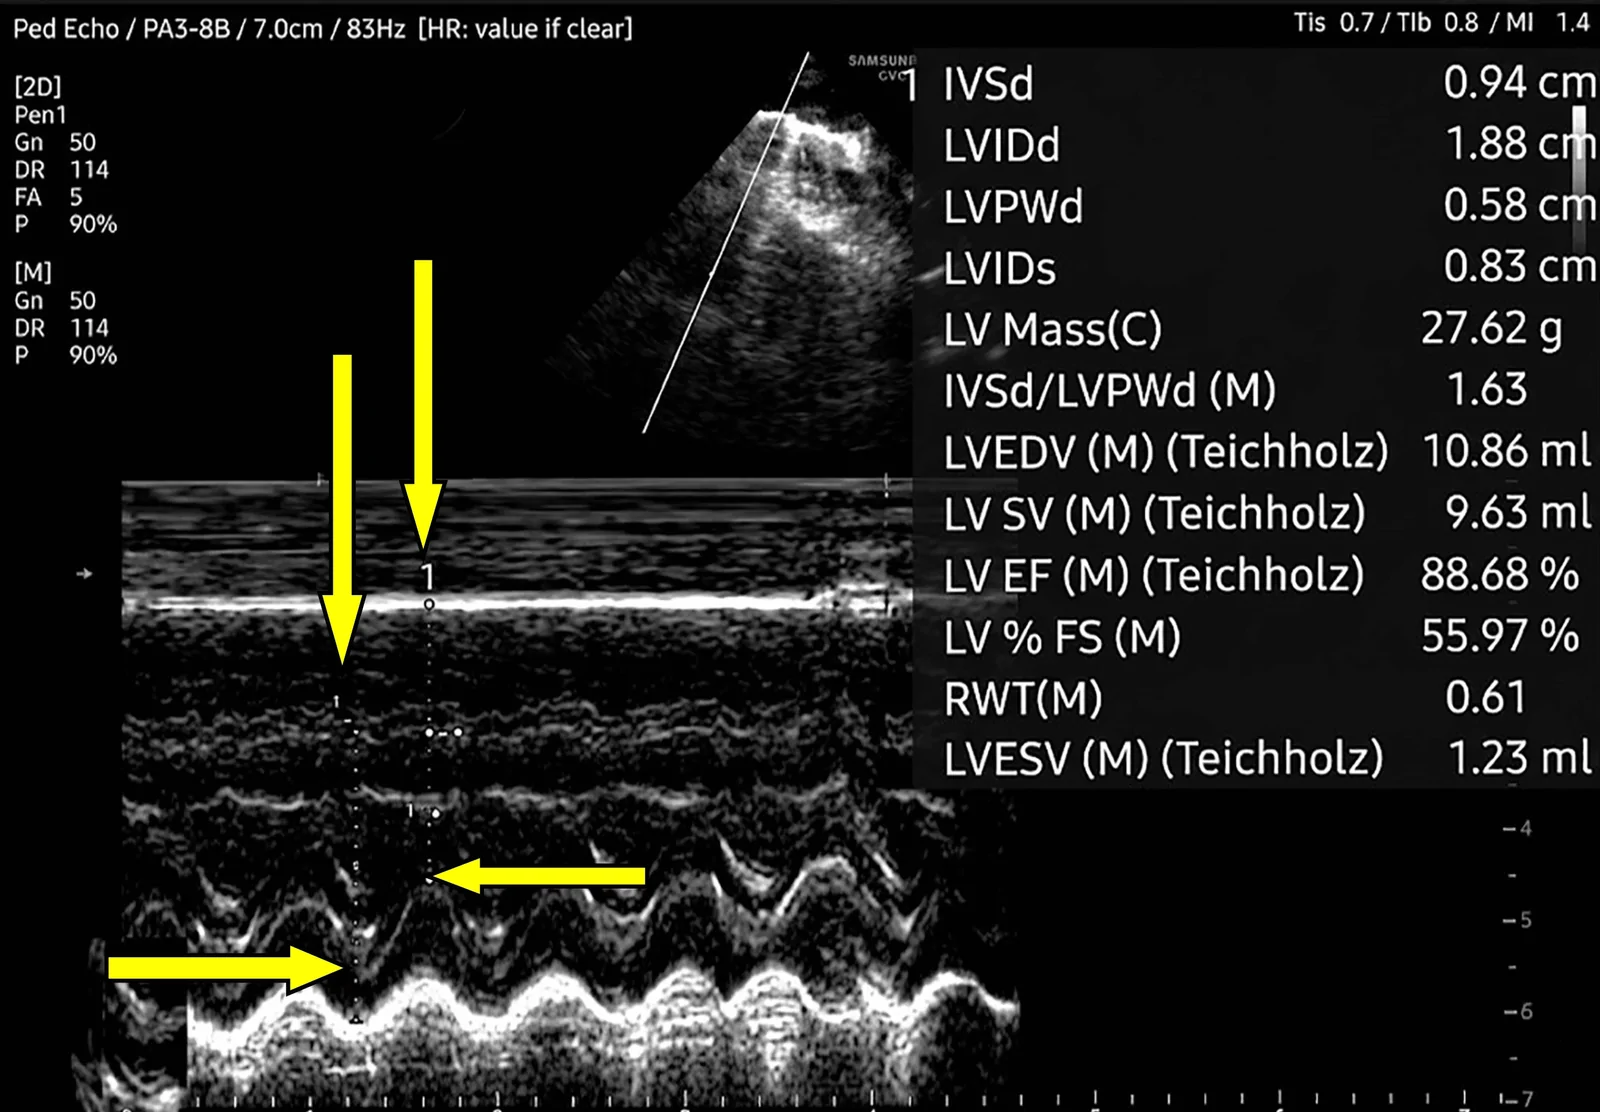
Postoperative M-mode echocardiographic evaluation of the left ventricle at three to four weeks follow-up. Parasternal short-axis M-mode view obtained three to four weeks postoperatively, demonstrating successful left ventricular adaptation to systemic workload. Measurement calipers (indicated by the yellow arrows) highlight a hyperdynamic left ventricle with an ejection fraction of 88.7% and fractional shortening of 56.0%. Diastolic interventricular septal thickness (IVSd: 0.49 cm/4.9 mm; indicated by the top caliper arrow) and diastolic left ventricular posterior wall thickness (LVPWd: 0.58 cm/5.8 mm; indicated by the bottom caliper arrow) confirm robust myocardial mass without regressive atrophy. EF, ejection fraction; FS, fractional shortening; SV, stroke volume; RWT, relative wall thickness; IVSd, diastolic interventricular septal thickness; LVIDd, diastolic left ventricular internal diameter; LVPWd, diastolic left ventricular posterior wall thickness.
